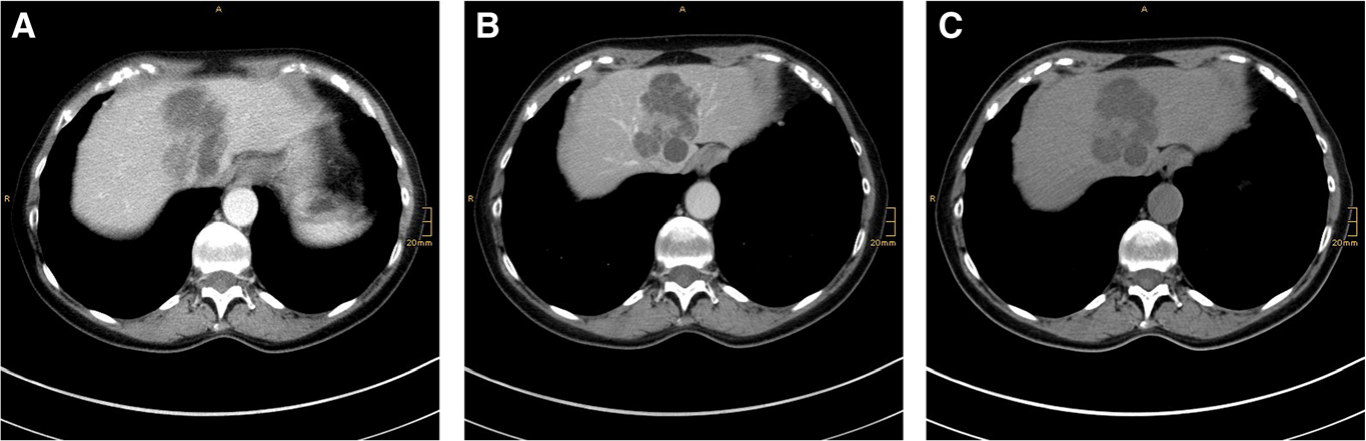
Radiologic findings. Axial CT image with contrast though the liver shows a cystic mass from segment IVa, with extension into segments II and IVb. a Hepatic arterial phase. b Portal venous phase. c Native
